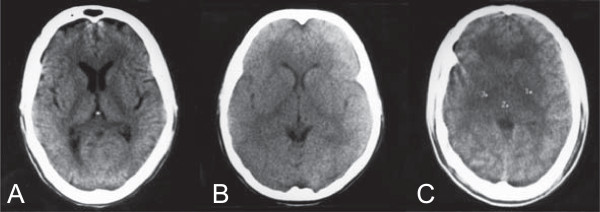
CT scans of adult CM patients from India showing mild to severe brain swelling. Non-enhanced 8 mm transverse sections of a control patient (A) and two acute CM patients showing mild and severe cerebral oedema (B and C, respectively). Images were obtained within 6 hours of admission at Ispat General Hospital in Rourkela, Orissa, India, as part of another study [52] and using a Philips W1000 third generation CT scan. Brain swelling observed in (B) and (C) was defined as loss of cerebrospinal fluid space, small ventricles, absence of sulci, and/or compression of the cisterns. Mild brain swelling was defined as local or diffuse effacement of sulci and Sylvian fissure without compression of the supratentorial ventricular system (B). Severe swelling was defined as diffuse obliteration of sulci, Sylvian fissure and basal cisterns, together with an important mass effect causing near total obliteration of the supratentorial ventricular system and generalized hypodensity of brain parenchyma (C).
